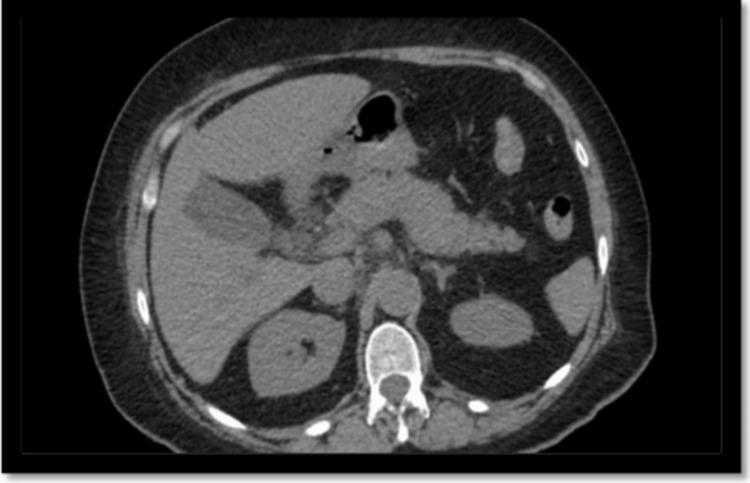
CT scan of the abdomen (with and without contrast): Mild fatty infiltration of liver. Partially calcified gallbladder wall. Mild perihepatic ascites and mild pericholecystic fluid. Mild diffuse peripancreatic stranding compatible with acute pancreatitis. No evidence of pseudocyst or abscess collections. Duodenal diverticulum. CT: computed tomography
